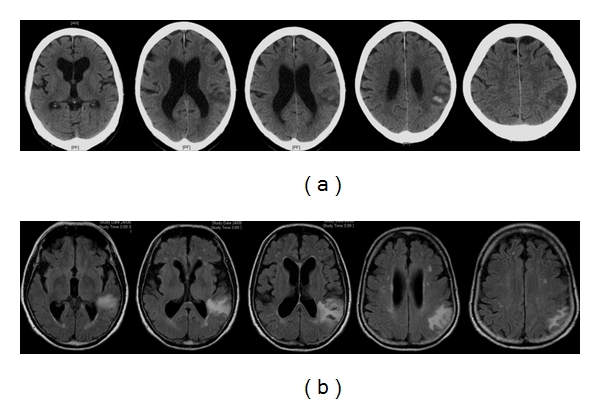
75-year-old man presented with slurred speech. (a) Axial unenhanced CT images show an acute left parietal hemorrhage. The hypodense area around the hemorrhage reaches superiorly and out to the cortical surface, following the middle cerebral artery territory. Stage HI c2 [1]. (b) Axial fluid-attenuated inversion recovery MR sequences confirm the area of infarction reaching the surface of the cortex, suggesting that the stroke is a hemorrhagic infarct.
